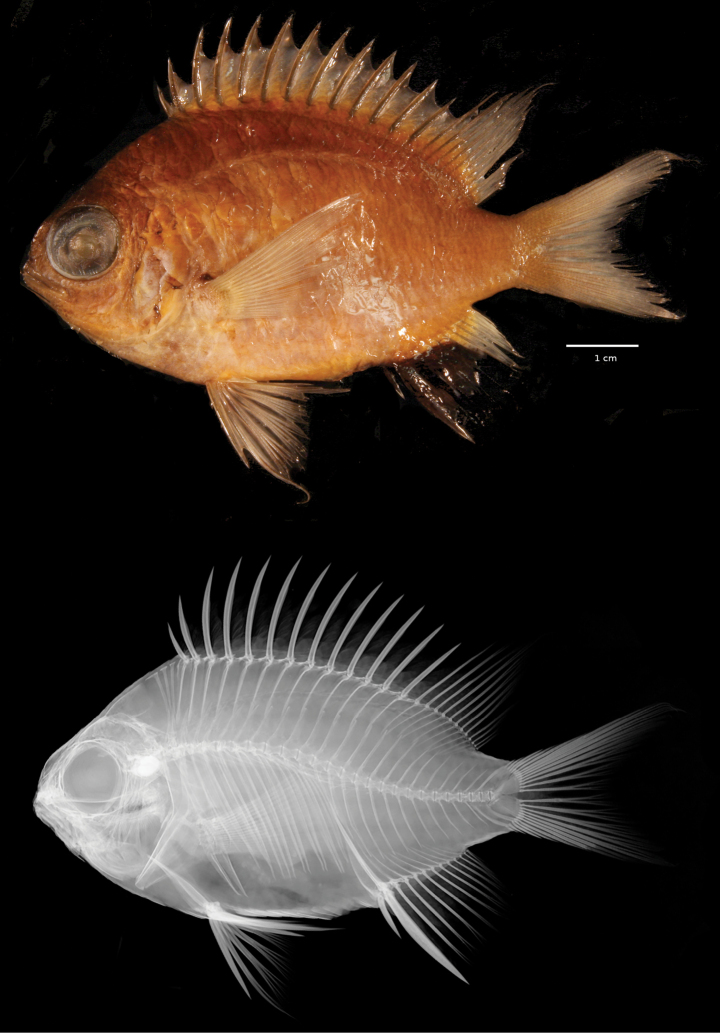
Preserved holotype and x-ray of *Chromisabadhah*, CAS 248401, 68.7 mm SL. Photo and x-ray by Jon Fong.
